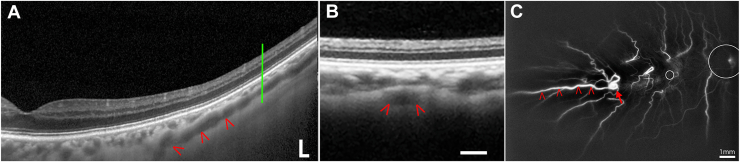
The OCT imaging of a long posterior ciliary artery (LPCA; arrowheads). A, Horizontal scan; B, vertical scan along the green line in A; note the periarterial halo (bars in A and B, 200 μm). C, Laser Doppler holography imaging of an LPCA (arrowheads). Two images were merged to achieve a 60° field of view. Note the focal arterial tortuosity (red arrow) at its emergence. Circles indicate the optic nerve and fovea (bar, 1 mm). See also Figure S1.
